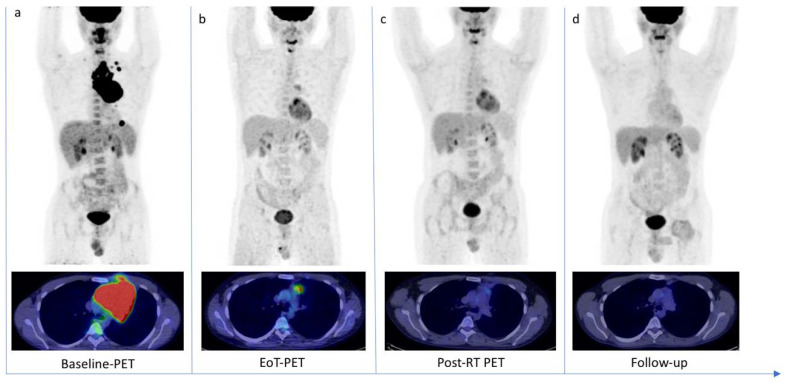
18F-FDG PET/CT scans of a 30-year-old patient with diffuse large B cell Lymphoma. (a) Baseline-PET showed an abnormal hypermetabolic mediastinal tumoral lesion (SUVmax 34.3) with supradiaphragmatic lymph nodes involvement (SUVmax 28.1). The patient underwent six cycles of chemotherapy. (b) The End-of-Treatment PET (EoT-PET) showed a partial metabolic response, with consistent morpho-metabolic disease reduction but persistent uptake in mediastinum (SUVmax 4.8): Deauville Score 4 was assigned and a Lesion-to-Liver Ratio of 1.45 was calculated. Patient underwent radiotherapy. (c) Post-radiotherapy PET (Post-RT PET) demonstrated a further reduction in tumor size and glucose metabolism (SUVmax 2.7). (d) After 10 months, a follow-up scan demonstrated a complete metabolic response (OS 25 months).
